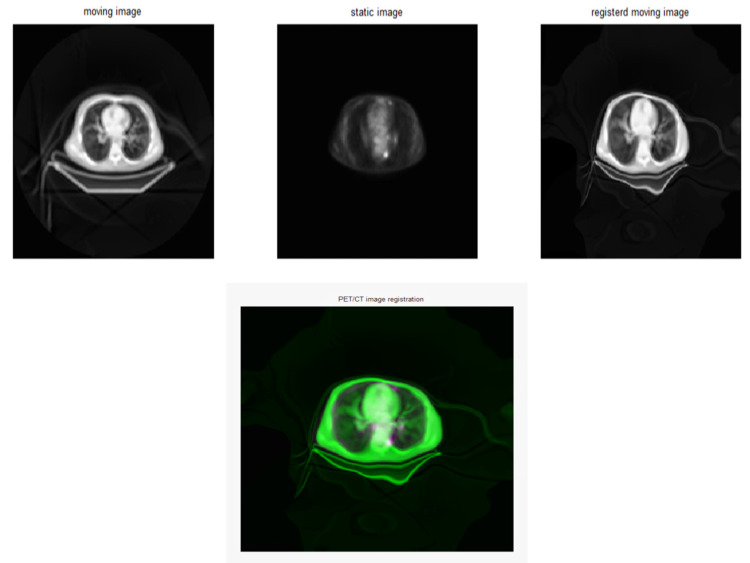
Example of Demons registration from LHS, with CT corresponding to the moving image and with PET as the static reference (n = 1 case shown) and registered moving image, when using a sigma fluid value = 4, a no. of bins = 256, and the contrast-stretching function adapthisteq. CT was selected as the fixed modality because of its superior spatial resolution, while PET was changed to CT to preserve anatomical accuracy.
